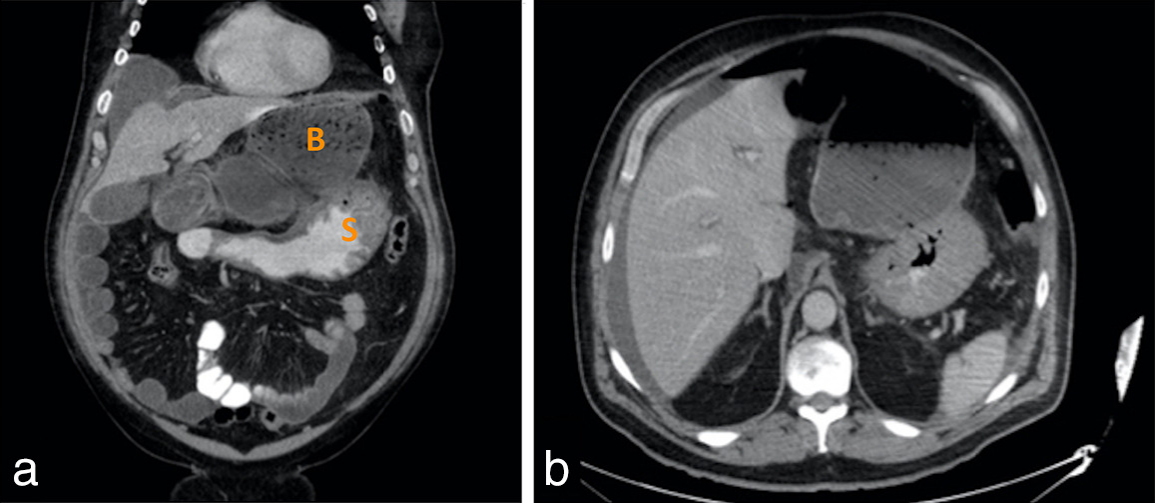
Contrast-enhanced CT scan of the abdomen and pelvis (day 3). (a) Coronal section demonstrating a grossly enlarged caecum and ascending colon (B) in the epigastrium, displacing the stomach (S) laterally and inferiorly; free fluid is also present. (b) Axial section showing the ascending colon in the lesser sac with free intra-abdominal air and fluid.
